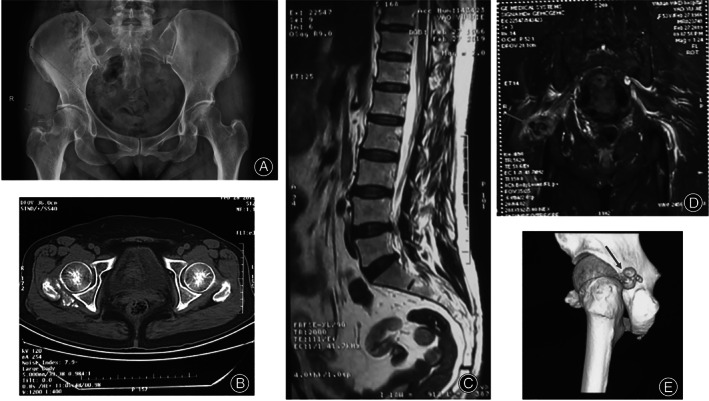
Preoperative imaging examination of the patient's lower back, pelvis and hip joint. (A) X‐ray examination of the pelvis in normal position; (B) CT of the right hip; (C) MRI of lumbar vertebrae; (D) MRI of hip; (E) three‐dimensional CT of the pelvis. In (A, B, and E) irregular cartilaginous bone and some nodular, posterior to the lateral side of the right hip joint, which adjacent to the greater trochanter of the right femur could be seen, as shown by the arrow. In (C) mild disc herniation in L_2‐3_, L_4‐5_, and L_5_‐S_1_ was observed, mainly L_5_‐S_1_ herniation accompanied by disc degeneration. In (D) abnormal signals in the superior and inferior gemellus regions of the right hip joint could be seen. Combined with CT results, synovial osteochondromatosis with peripheral soft tissue edema was considered.
